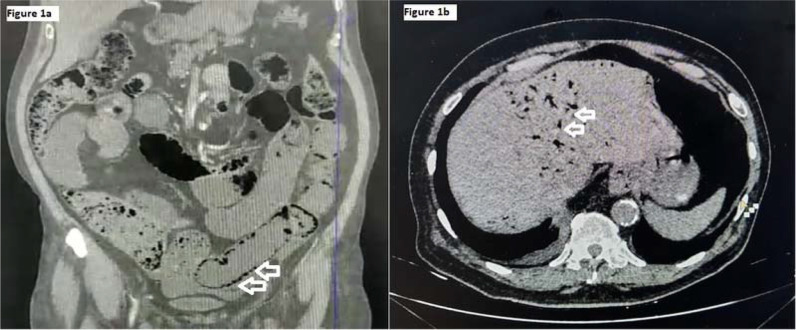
Computed tomography angiography of the abdomen showing pneumatosis intestinalis (Fig. 1A) and air in the portal vein (Fig. 1B) (marked with white arrows)
